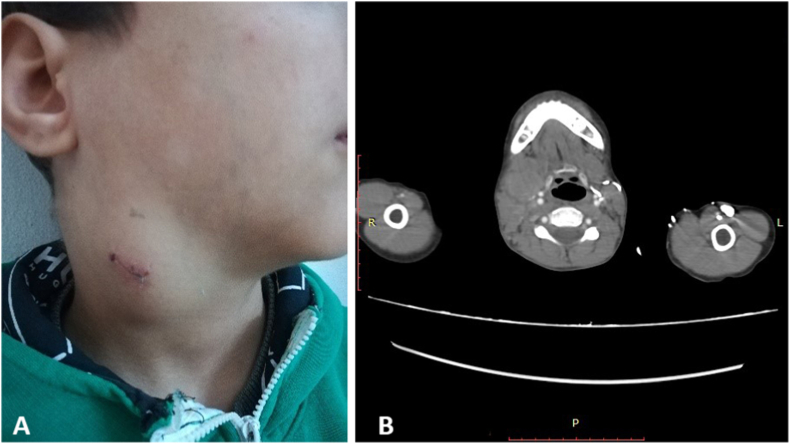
(A): Clinical view of the patient showing right lateral cervical mass measuring approximately (3 × 5) cm. (B): CT scan in axial section revealing enlarged lymph nodes measuring approximately (44x38)mm on the right side of the neck.
